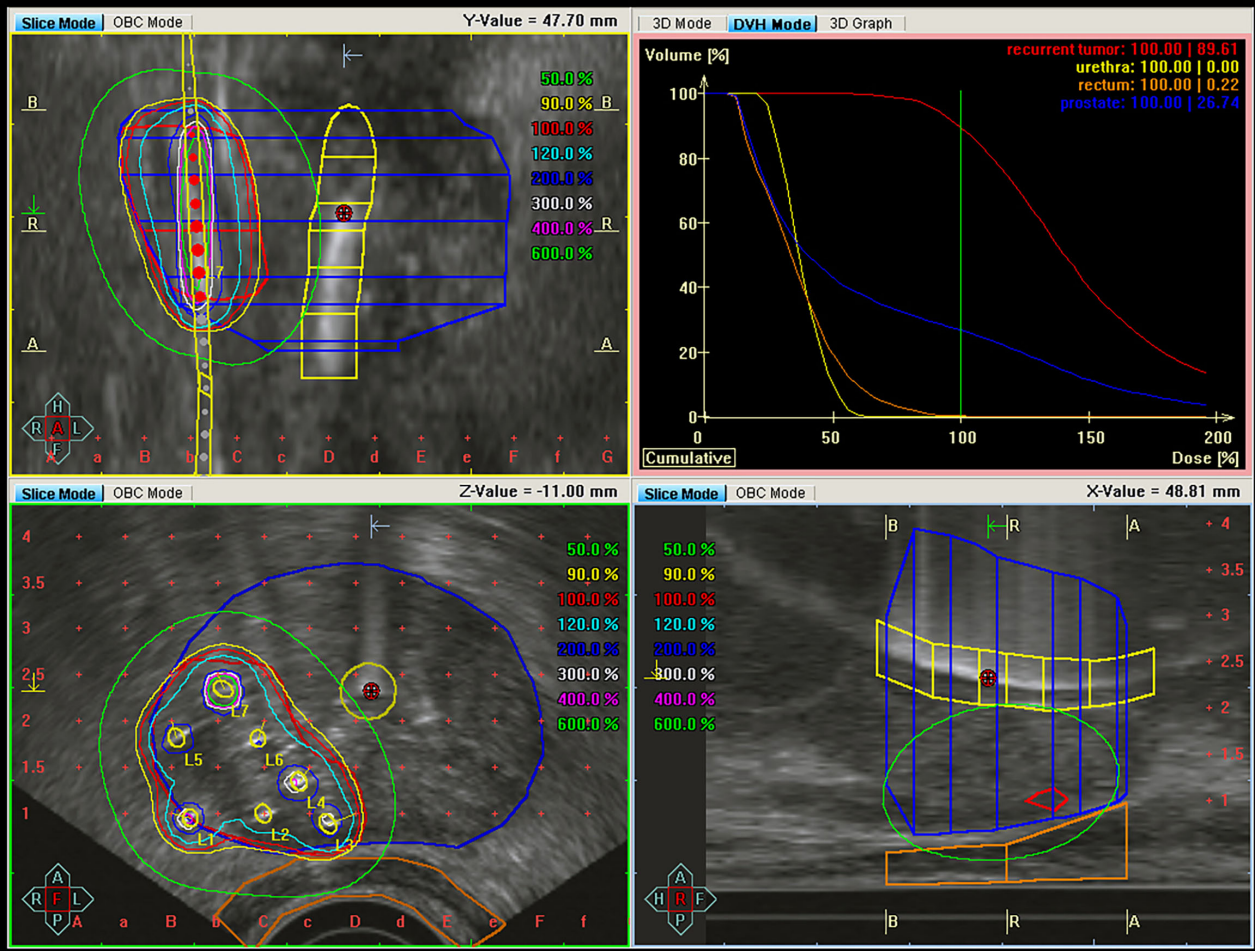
Dose distribution for high dose-rate brachytherapy of the same patient as in Figure 2 showing the coronal (upper left), transversal (lower left), and sagittal (lower right) plane. The recurrent tumor (red), prostate (blue), urethra (yellow), and rectum wall (brown) are delineated. Air-filled gel has been inserted in the urethra catheter to visualize the urethra in the ultrasound images. The dose-volume histograms (upper right quadrant) show the highly conformal dose distribution, sparing the rectum and urethra, achieved by brachytherapy.
